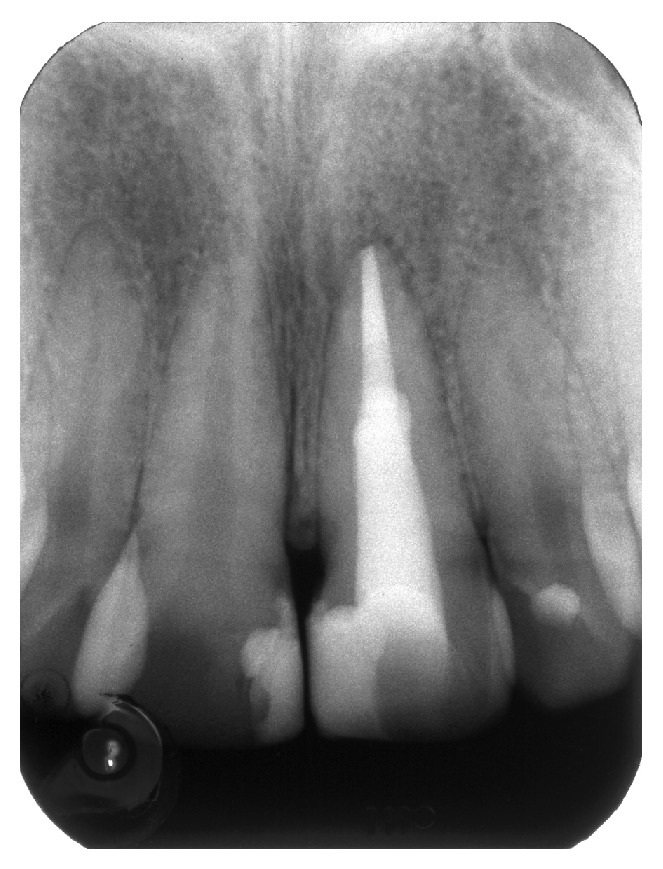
Radiograph at 3 months after surgery.
